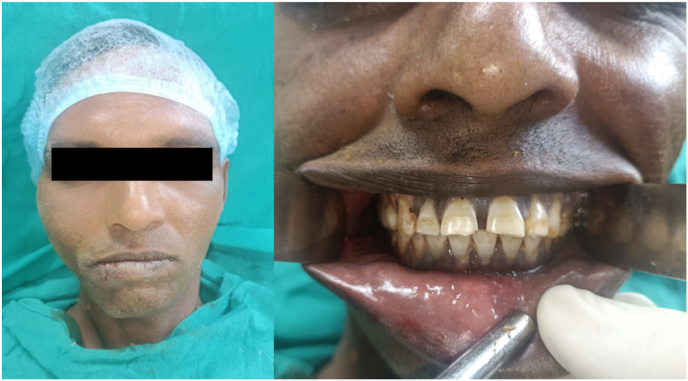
Postoperative CT scan (or panoramic X-ray) confirming anatomic reduction of the zygomatic arch (arrow) with the coronoid process returned to its normal position beneath the arch.
